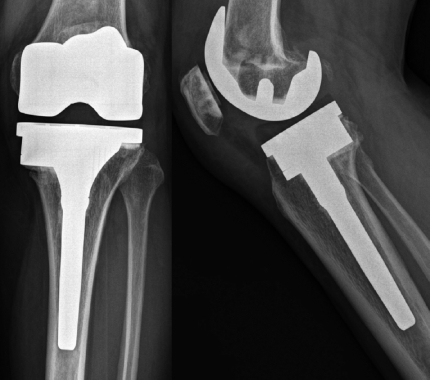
Immediate postoperative radiographs of knee replacement with double metal blocks.
